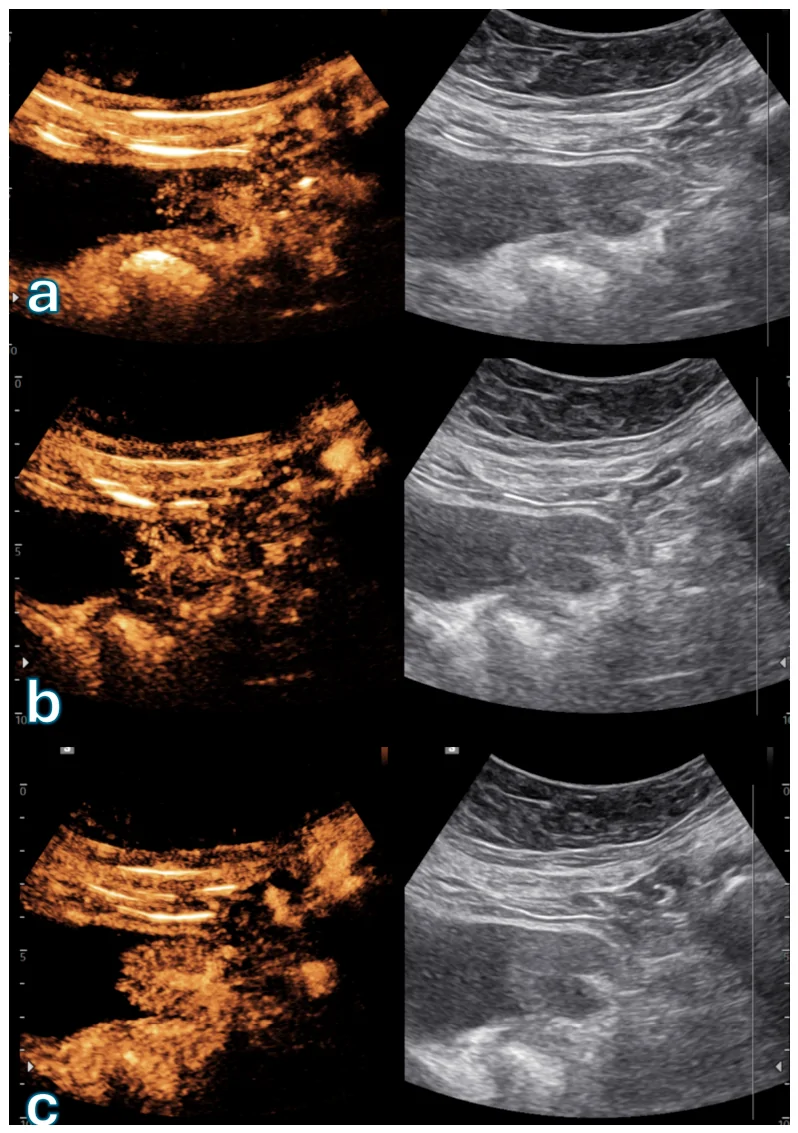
Real-time CEUS demonstration of the vascular stalk sign in a papillary bladder tumor. Split-screen images show contrast-enhanced ultrasonography on the left and the corresponding gray-scale image on the right. Successive frames obtained during contrast wash-in demonstrate initial enhancement of a thin vascular pedicle arising from the bladder wall while the tumor remains largely unenhanced (a), followed by progressive propagation of enhancement from the pedicle into the lesion (b) and subsequent more diffuse enhancement of the tumor (c). This temporal sequence fulfilled the predefined criteria for a positive CEUS vascular stalk sign.
